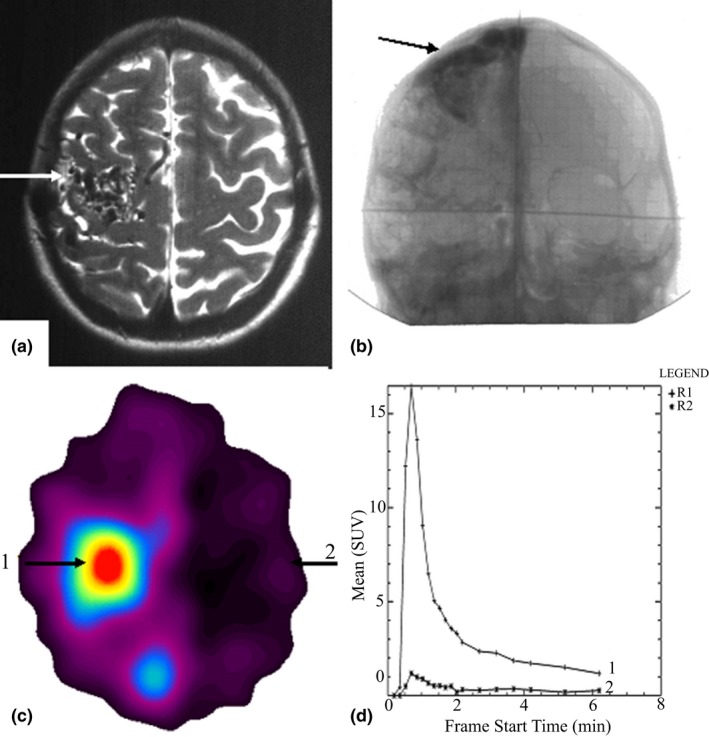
(a‐d) Female patient Z. Diagnosis: arteriovenous malformation of the right parietal lobe. (a) MRI (T2‐weighted image) showing that AVM is well visible. (b) The angiography (in sagittal slice) shows that AVM is well visible, a developed vast vasculature of the aneurysm is observed. (c) Under the ^82^Rb‐chloride PET examination, we observed a heterogeneous hypervascular focus (position 1), that was swell‐visualized within the tissue phase, but the radiopharmaceutical uptake within the vascular phase was low (UI = 1.1–1.2). Contralateral brain cortex is shown in position 2. (d) Activity/Time curve of AVM shows that the malformation has high vascularization degree (VD = 14), but radiopharmaceutical rapid biexponential clearance took place in the tissue phase. The activity/time curve (2) over contralateral cortex is shown for comparison
